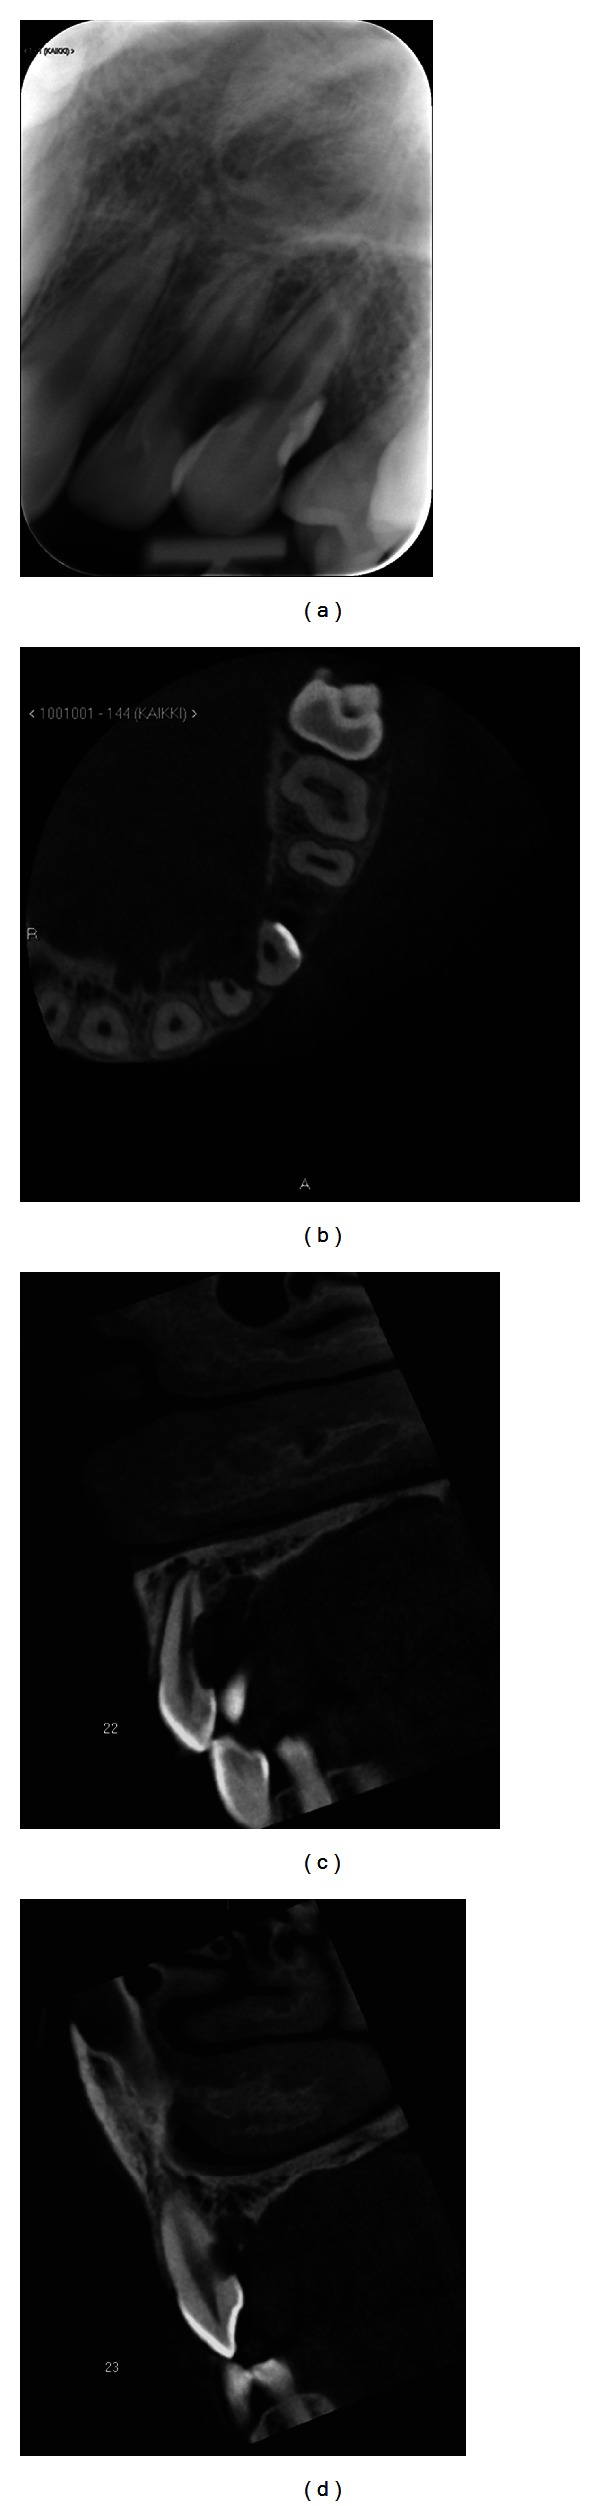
(a) The root resorption is visible in the intraoral X-ray of 22-23. (b) CBCT axial slice above the marginal bone line. A 6 × 10 mm bone destruction, which is sharply lined, is visible in the region 22-23 on the palatinal side. The roots of the teeth 22-23 have resorption on the palatinal sides. (c) CBCT sagittal slice presenting the root of 22. On palatinal side of the root, there is a 2 × 4 mm deep resorption defect. Lesion on palatinal bone structure can also be seen. (d) CBCT sagittal slice of 23.
